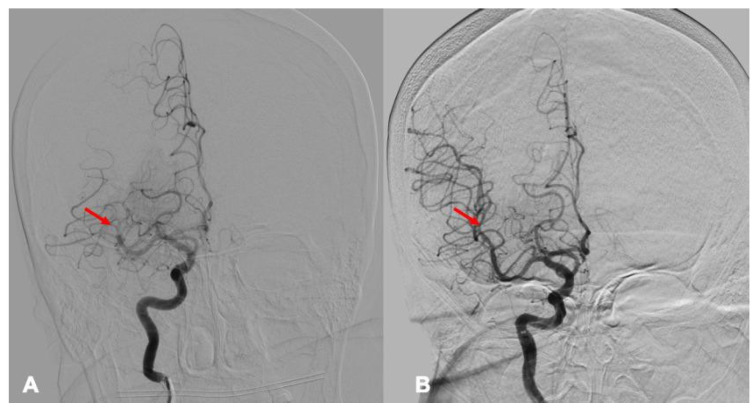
Patient case example: Mechanical thrombectomy evaluation. In the same patient, digital subtraction angiography (DSA) did not reveal visible occlusion in the right MCA M1 segment, previously identified on CTA, indicating spontaneous recanalization. However, an occlusion in the M2 segment (A, red arrow) was detected and treated with mechanical thrombectomy. A total of four passes were performed, achieving a reperfusion level of mTICI 2C (B). The duration of the procedure was 43 min and the post-procedure NIHSS score was 1 point.
